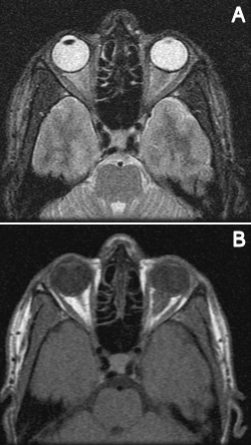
Magnetic resonance imaging showing a retrobulbar orbital mass in the left orbit
